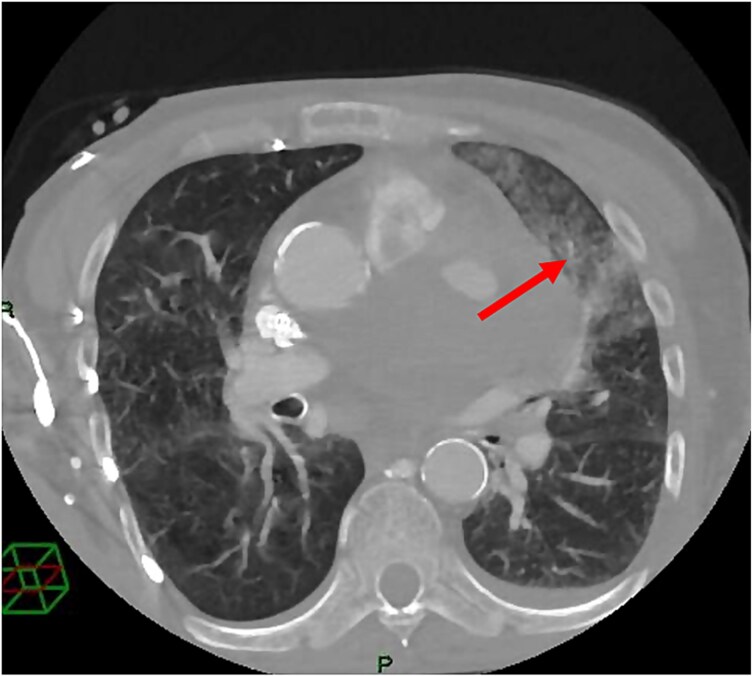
CT scan performed after re-admission for septic status. Area of alveolar haemorrhage (arrow).
